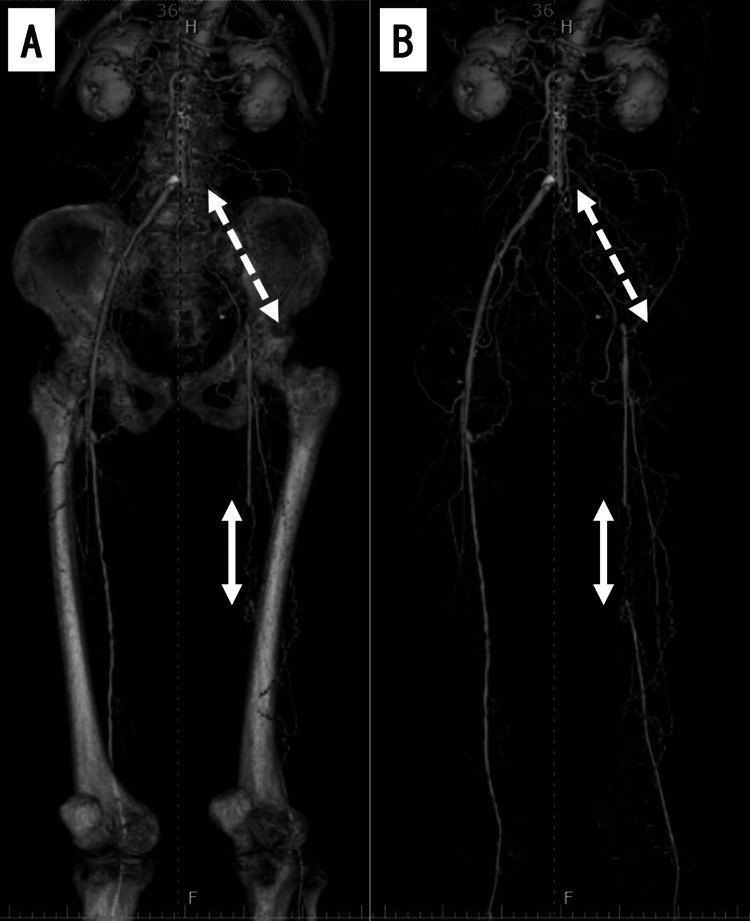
Three-dimensional computed tomography angiography of lower extremity arteries. (A) Image with bones. (B) Image without bones. These images showed complete occlusion of the left common iliac artery to the external iliac artery (white dotted arrow) and complete occlusion of the left superficial femoral artery (white arrow).
